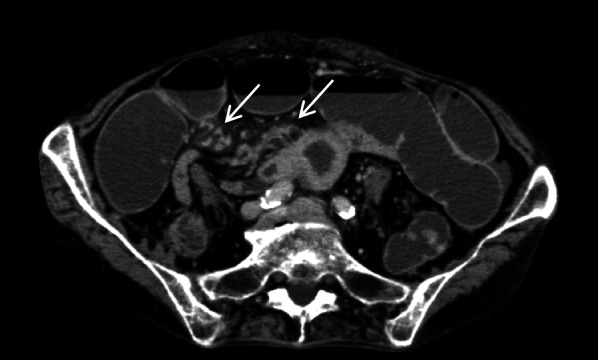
The enlarged and winding peripheral marginal veins are observed on computed tomography (white arrow)
